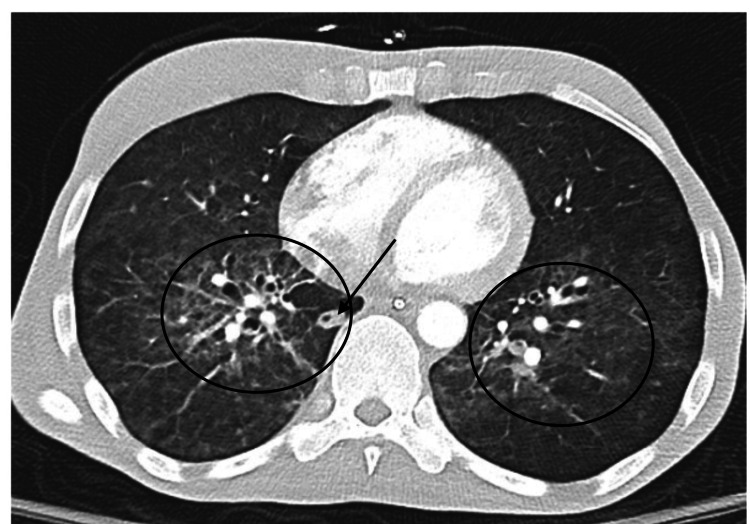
CT of chest at admission showing bilaterally ground-glass opacities and septal thickening (circles) with central cavitation (arrow)
